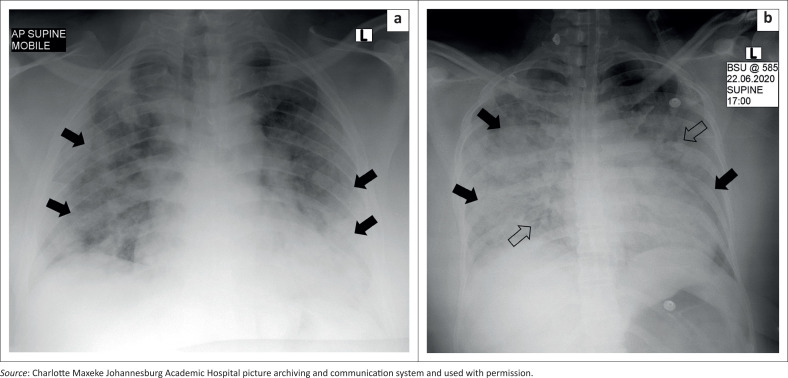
(a) Chest radiograph of a 39-year-old man with type 2 diabetes mellitus and hypertension who presented with a cough, dyspnoea and fever. There are typical findings of predominantly peripheral, patchy ground glass opacities bilaterally (black arrows). (b) Chest radiograph of a 48-year-old woman with asthma, hypertension and severe COVID-19 pneumonia. Bilateral, multi-zonal, patchy, ground glass opacities (black arrows) and consolidation with air bronchograms (open arrows).
